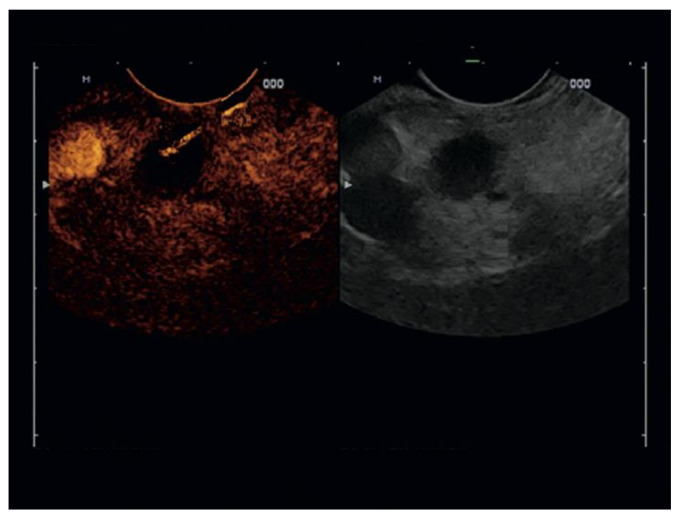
Endoscopic ultrasound contrast-enhanced fine-needle aspiration of a pancreatic adenocarcinoma. The hypoenhancement area of the lesion was selected as a target area for fine-needle aspiration.
